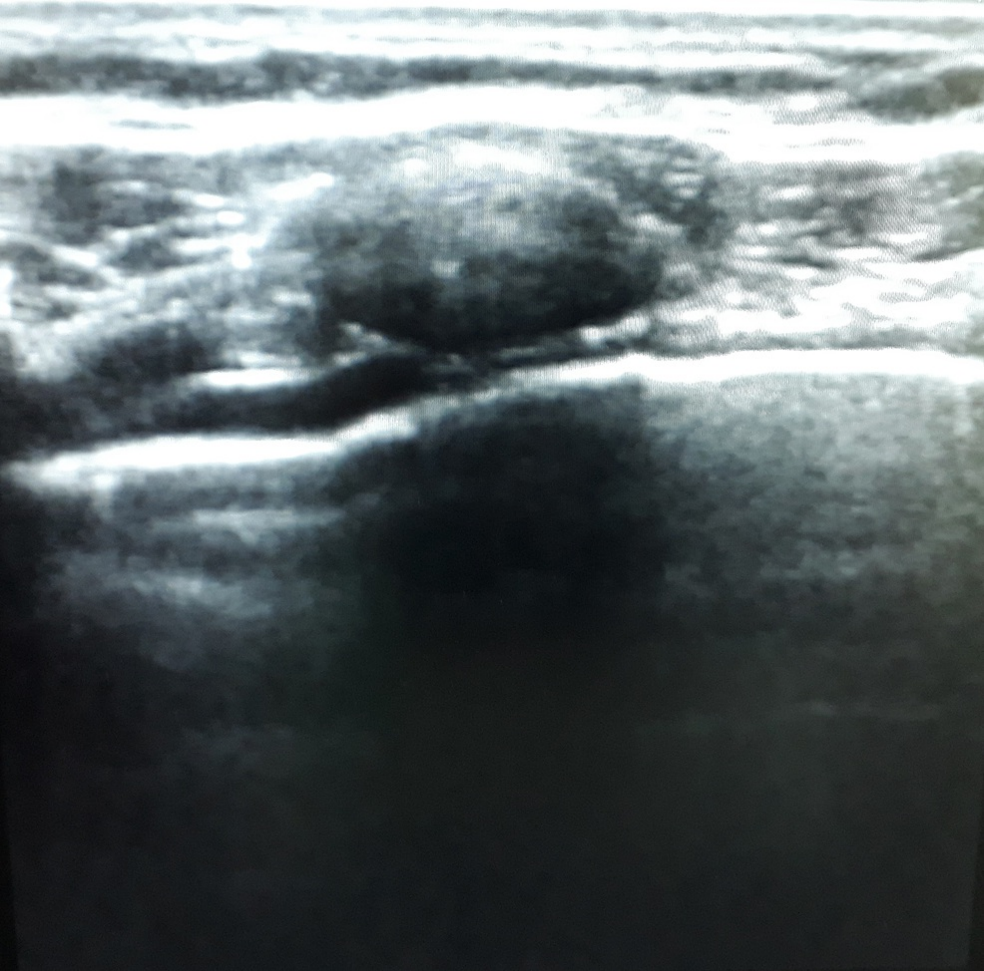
Lung ultrasound image showing minimal amount of air between the lung parenchyma and the chest wall
